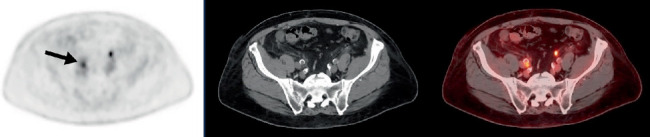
A single suspected nodal metastasis from prostate cancer was present in the pelvis, with abnormal PSMA uptake (*black arrow*; SUVmax 4.0) localizing to a 4-mm lymph node posterior to the right external iliac artery.
